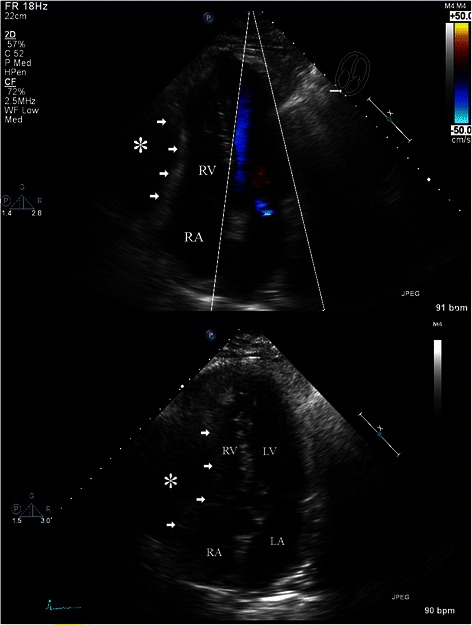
Apical Four Chamber Views Transthoracic Echocardiogram. These apical four chamber views on transthoracic echocardiogram demonstrate extensive extra-pericardial accumulation of blood and collapse of the right ventricle. Arrows: Compression of right ventricle, Asterisk: Hematoma, RA: Right atrium, RV: Right ventricle, LA: Left atrium, LV: Left ventricle
